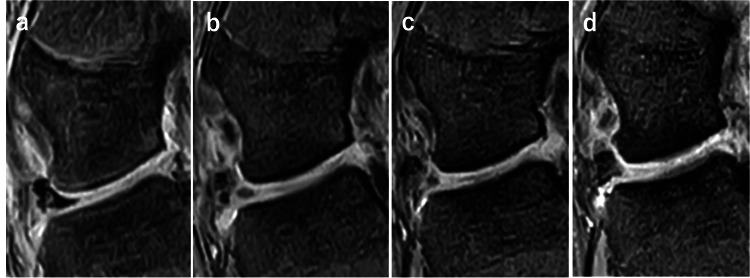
The change over time of the tendon graft on the coronal view of MRI (a) Post-operative at 10 days; (b) post-operative at three months; (c) post-operative at six months; (d) post-operative at two years.
